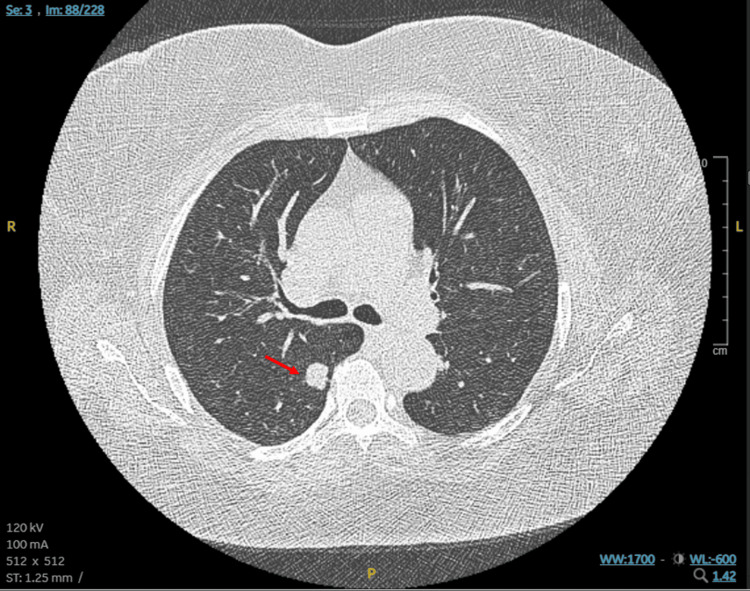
Low-dose computed tomography scan (LDCT) of the chest. Low-dose CT (LDCT) of the chest (axial view) demonstrating a 1.6 cm solitary pulmonary nodule (red arrow) in the superior segment of the right lower lobe. Note the complete absence of associated central airway involvement or hilar/mediastinal lymphadenopathy, which is an atypical presentation for small cell lung cancer.
